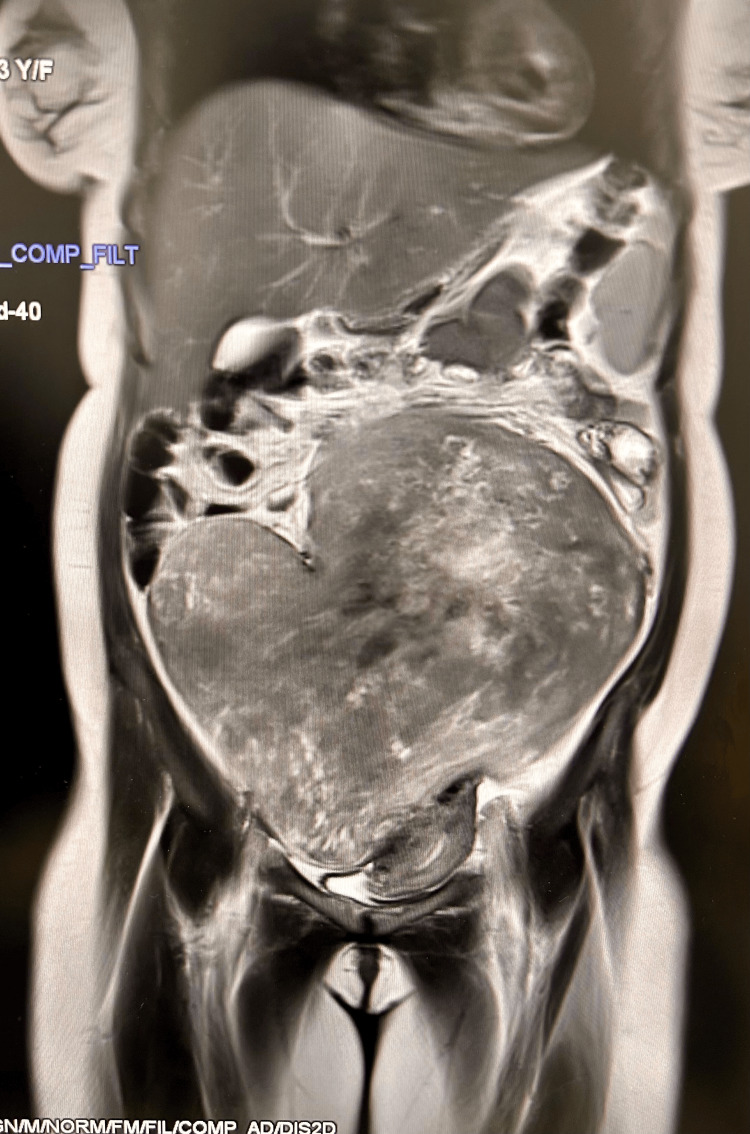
Case 1: MRI pelvis T2 sequence showing 13 × 20 × 22.5 cm mass A heterogeneously isointense mass arising from the right parametrium fills the entire pelvis, displacing the uterus to the left. MRI findings were suggestive of neoplastic aetiology, driving the decision for intraoperative frozen section.
